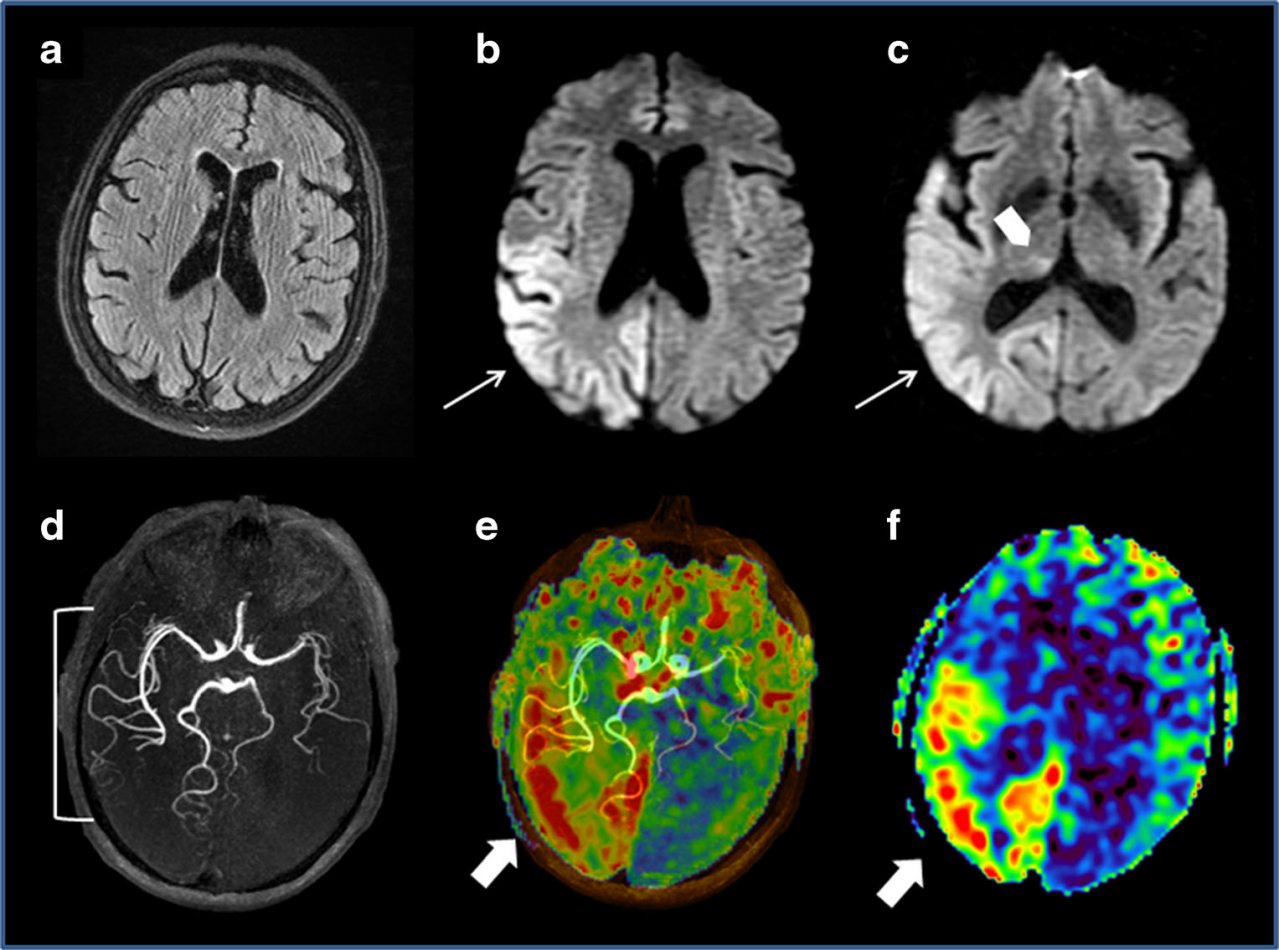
Brain MRI in a 65-year-old patient with sudden-onset left hemibody deficit. FLAIR (a), DWI (b and c), 3D TOF (d), ASL/TOF fusion (e), and ASL (f) sequences in the axial plane. No visible lesion on the FLAIR sequence. b and d Hyperintense cortical zone on the DWI images showing a right temporo-parieto-occipital zone not corresponding to a vascular territory (*white arrow*), with involvement of the homolateral pulvinar (*arrowhead*). d, e and f Dilatation of the sylvian and right posterior cerebral arteries (*parentheses*) associated with elevated CBF (*white arrows*) in a context of status epilepticus
